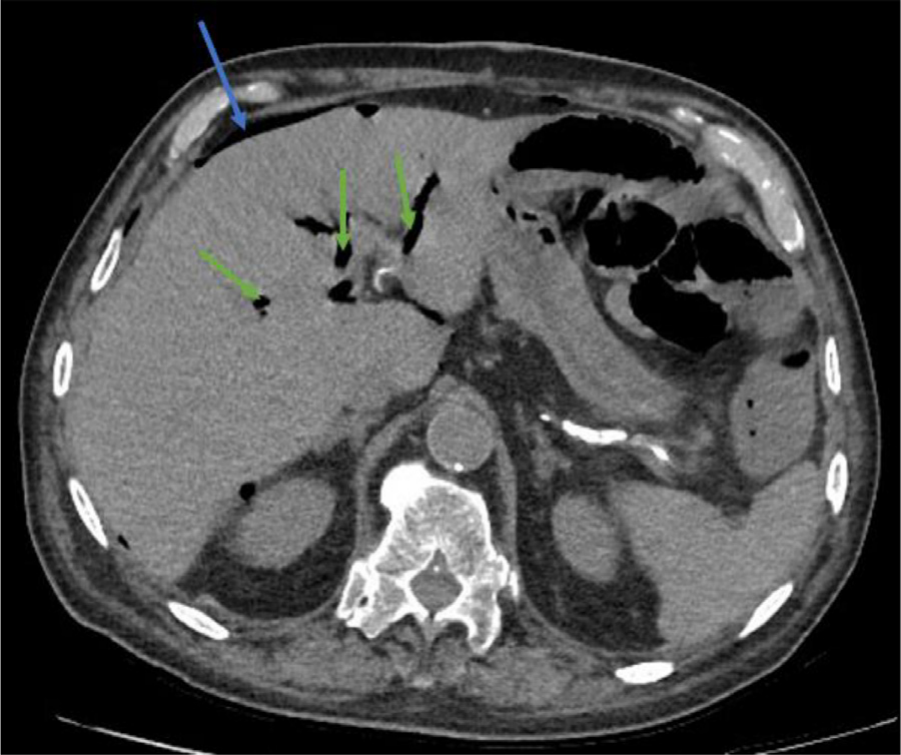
Noncontrast phase abdominal CT showing subdiaphragmatic free air (blue arrow), and air collection within the intrahepatic biliary tree.
